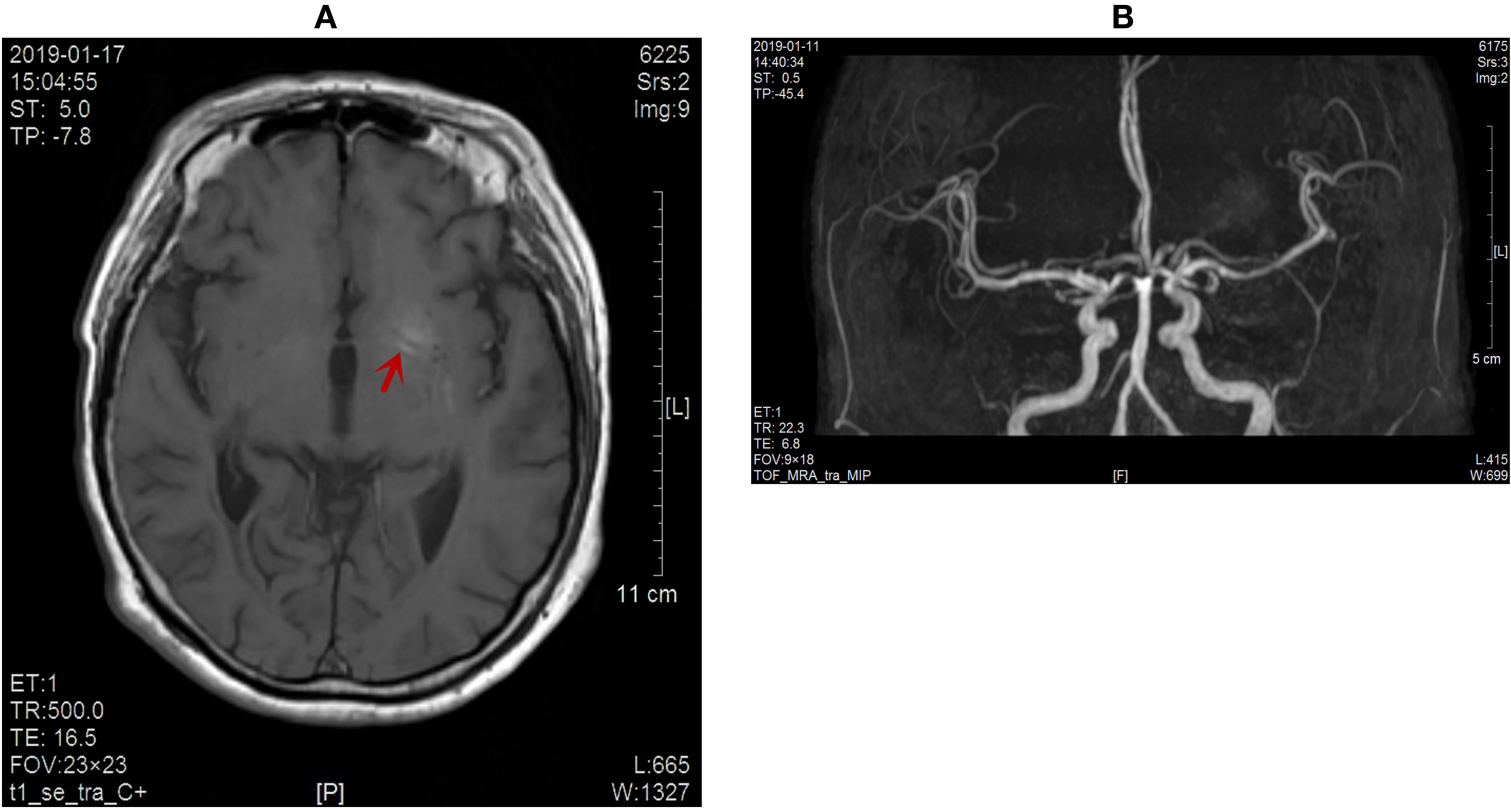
(A) No obvious enhancement of the lesion on contrast-enhanced MR images (red arrow). (B) No abnormal blood vessel shadow observed on the skull MRA scans.
